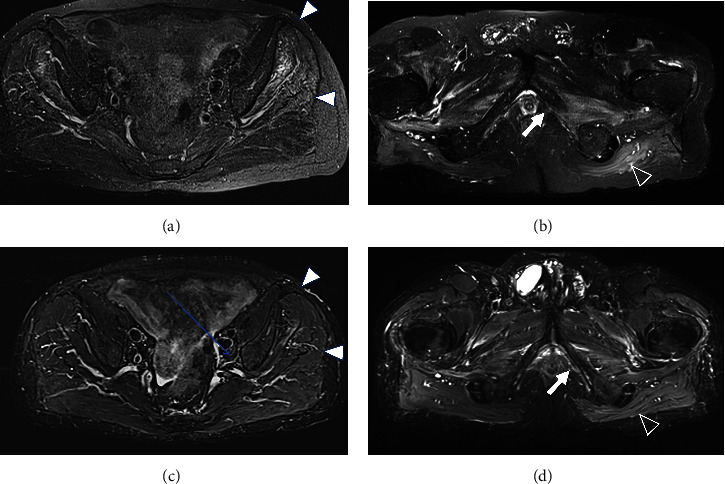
MRI of skeletal muscle. The figure shows four MRI axial views of pelvic girdle muscle acquired before (a, b) and after (c, d) therapy. The images are T2-weighted sequences with chemical-shift fat saturation obtained at the level of greater sciatic foramen (a) and (c) and at the level of ischial tuberosity (b) and (d). In (a), mild hyperintensity of signal within the muscles gluteus minimun and medium (arrowhead) on the left side can be observed, and it is related to intramuscular edema. After therapy, the edema is significantly reduced (arrowhead in (c)). Similarly, in the more caudal scan, the edema related to the inflammatory process can be appreciated in the left obturator externus, adductor magnus muscles (arrow in (b)) and left gluteus maximum muscle (void arrowhead in (b)). After therapy, the intramuscular edema of obturator externus and adductor magnus is less evident (arrow in (d)) while it is completely resolved in the gluteus maximus (void arrowhead in (d)). The muscle pathologic signal alterations are bilateral but on the right side they result more faint, keeping with the patchy and asymmetrical muscular involvement of the statin-induce myonecrosis.
